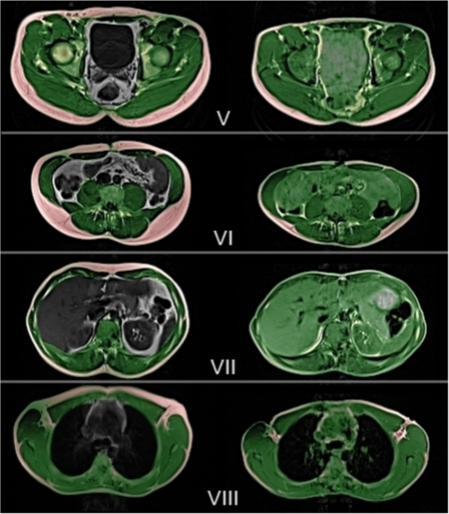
Semiautomatic separation of somatic and visceral volume (right row) and intraluminal nutrition fat (left row): selected slices from whole body MRI of a 32-year-old male finisher of TEFR09. V: hip/pelvis, VI: umbilical level, VII: upper abdomen, VIII: heart/mediastinum. Left row: before start (t0), green: SLT, red: TSAT, grey: TVV. Right row: after 4,120 km of running (t5), green: TLT, red: SAT (=SAST+ABM), yellow: VAT, blue: INF. ABM, adipose bone marrow; INF, intraluminal nutrition fat; MRI, magnetic resonance imaging; SAT, somatic adipose tissue; SLT, somatic lean tissue; TEFR09, Transeurope Footrace 2009; TLT, total lean tissue; TSAT, total somatic adipose tissue; TVV, total visceral volume; VAT, visceral adipose tissue.
